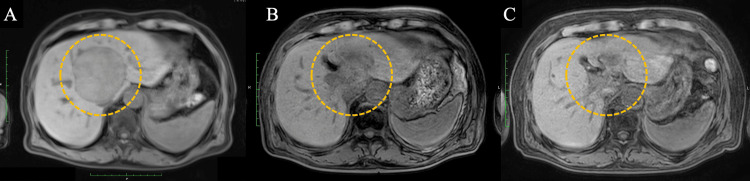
Serial imaging changes of hepatocellular carcinoma after proton beam therapy (A) Pre-treatment MRI shows a 70-mm hepatocellular carcinoma in hepatic segment 1 (dotted circle). (B) MRI at one year after proton beam therapy demonstrates lesion shrinkage with indistinct lesion margins (dotted circle). (C) MRI at two years after proton beam therapy shows complete disappearance of the lesion (dotted circle).
